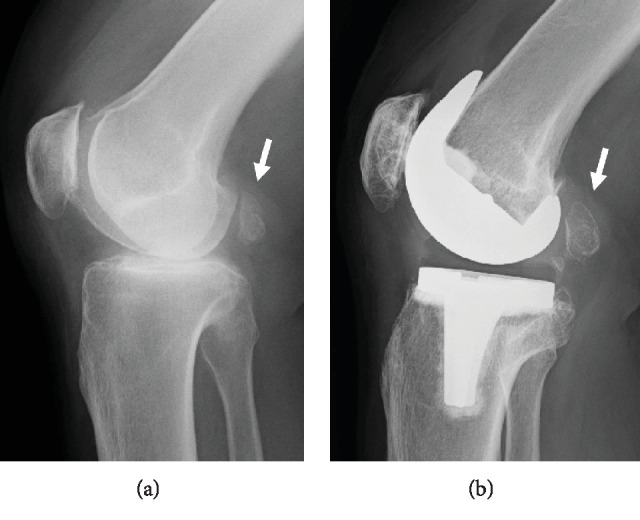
(a) Radiograph before TKA. (b) Enlargement of the fabella (arrow) is shown on radiograph 8 years after TKA.
